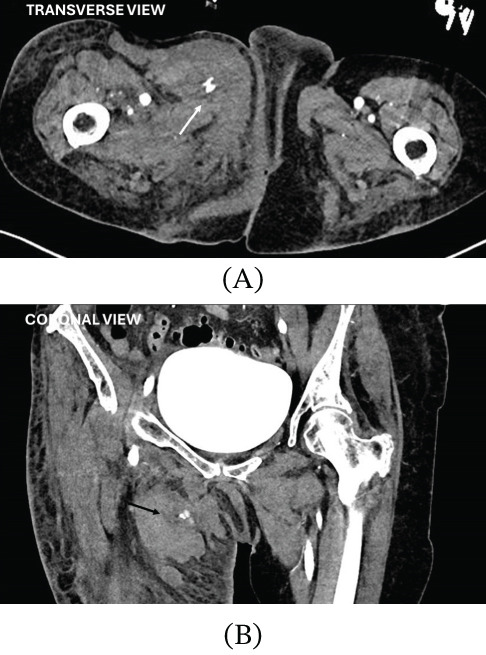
CT angiography demonstrating an extensive right groin haematoma with active contrast extravasation. (A) Transverse view: White arrow indicates active contrast extravasation within the haematoma, which extends medially along fascial planes towards the perineum. (B) Coronal view: Black arrow demonstrates a large haematoma (approximately 8 × 7 cm) within the right femoral triangle, with medial tracking into the vulval region.
